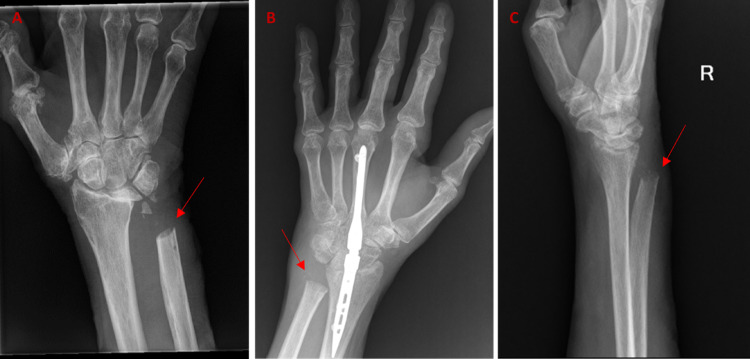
Sharp Edges of the Distal Ulna. (A) An 81-year-old male, a two-month post-operative image presenting with an inability to extend ring finger and small finger, was found to have extensor tendon rupture confirmed by MRI. Red arrows denote a sharp edge of the distal ulna in all images. (B) A 63-year-old female, two-month post-operative image, presenting with an inability to extend small finger and weak ring finger extension. (C) A 68-year-old female, four-week postoperative imaging, presenting with the loss of ring finger extension.
